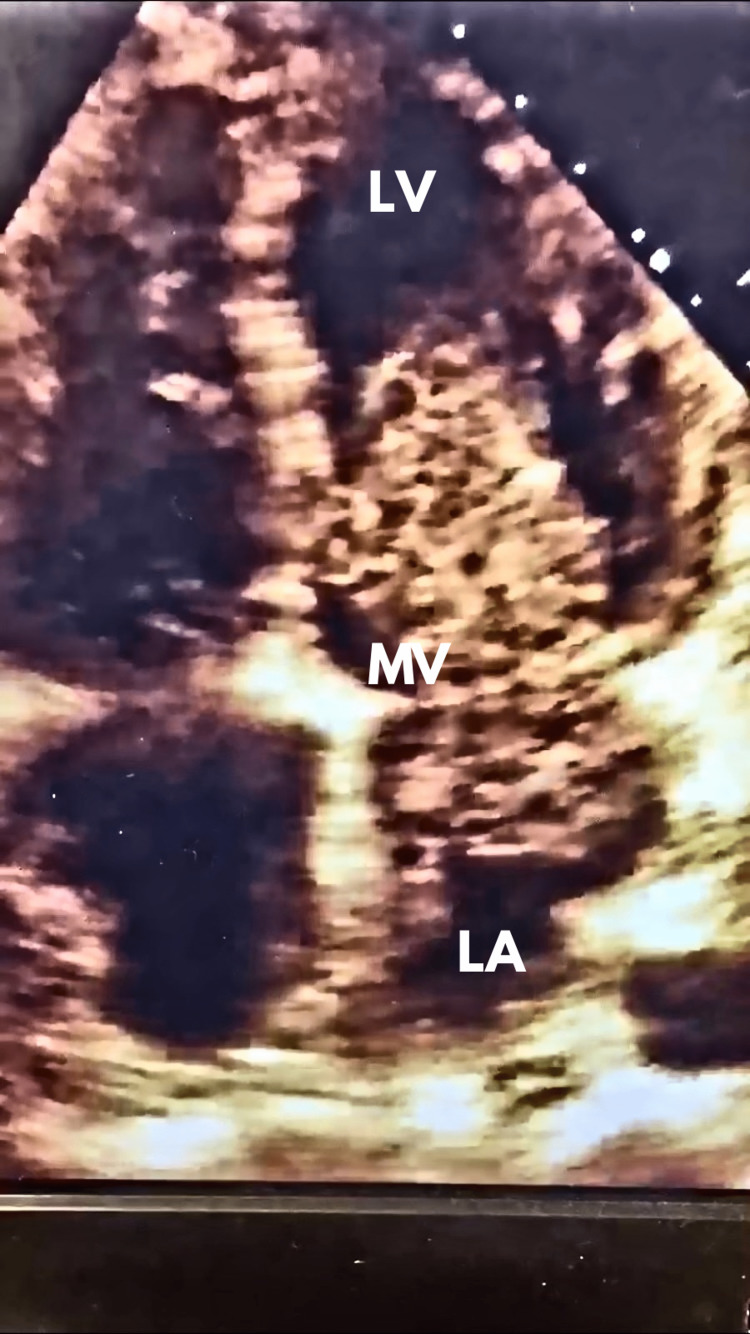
Photograph of TTE demonstrating myxoma protruding through the patient’s mitral valve. LA: left atrium; LV: left ventricle: MV: mitral valve; TTE: transthoracic echocardiogram
